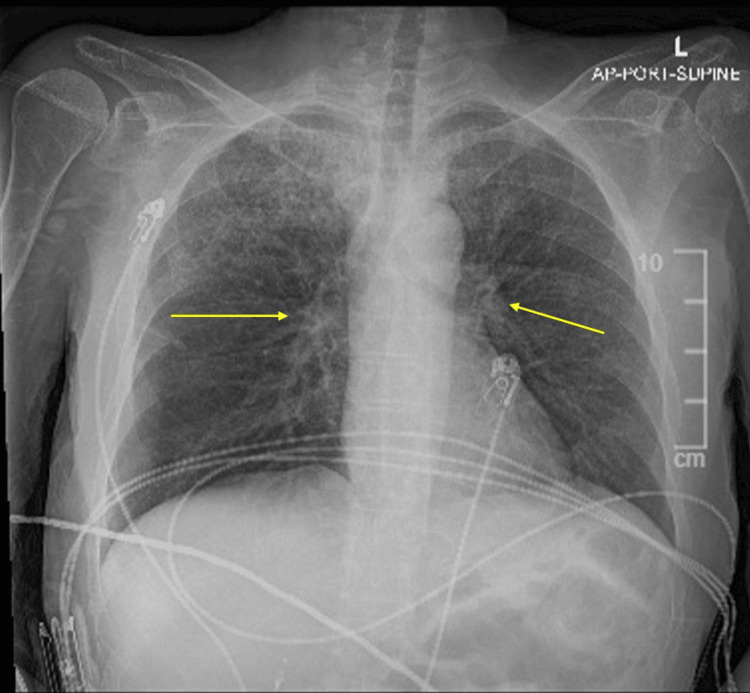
Chest X-ray The patient's chest X-ray showed minimal diffuse interstitial opacities suggestive of atypical pneumonia
